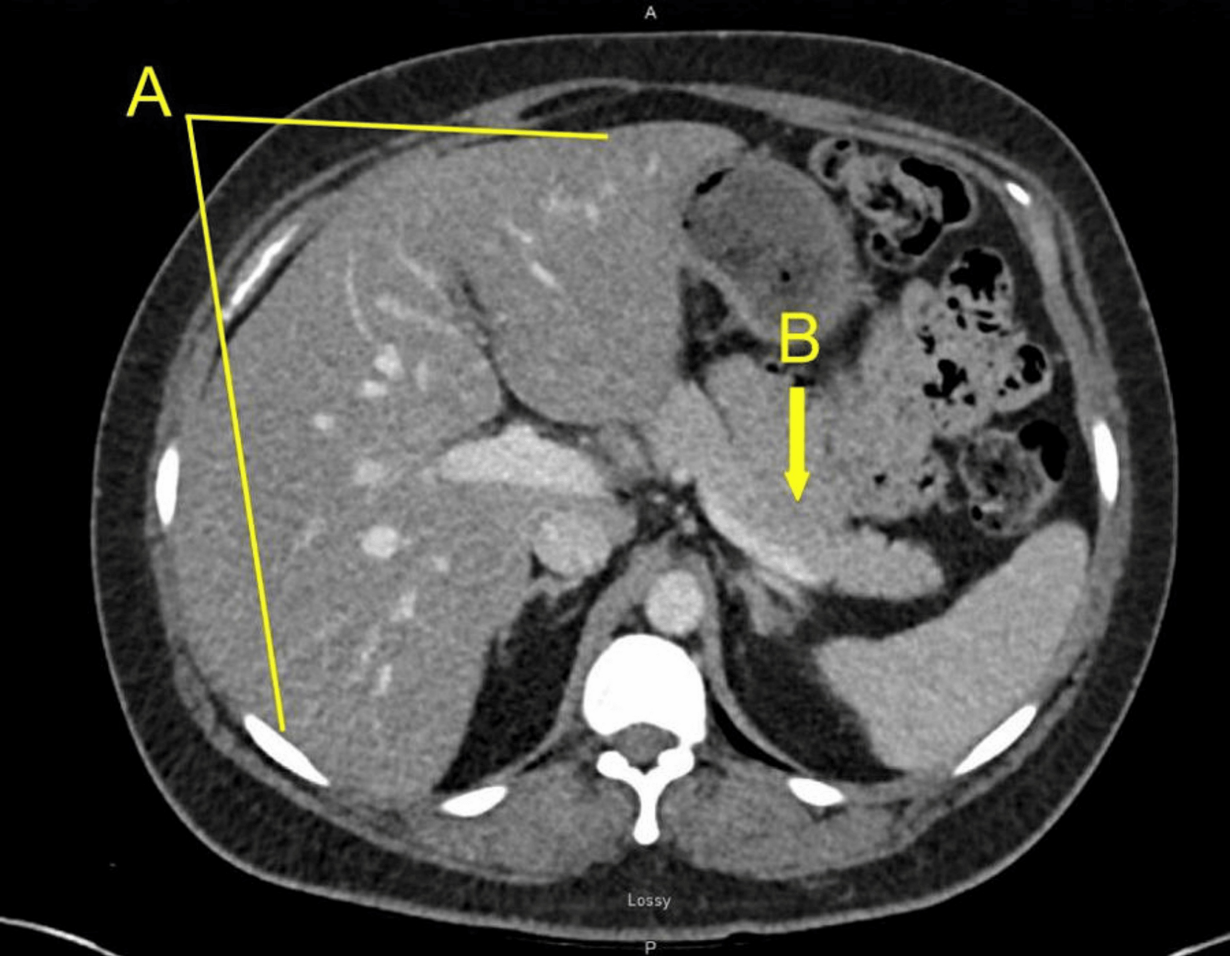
Abdominal CT Without Evidence of Acute Pancreatitis Despite Severe Hypertriglyceridemia (>4,000 mg/dL) Low-density liver (A) enlarged and hypodense compared to spleen, consistent with hepatic steatosis. No acute pancreatitis changes visible on this slice; though evaluation of pancreas (B) requires multiphase review. No focal hepatic or splenic lesion, no free fluid, no obvious acute intra-abdominal pathology in this cut.
